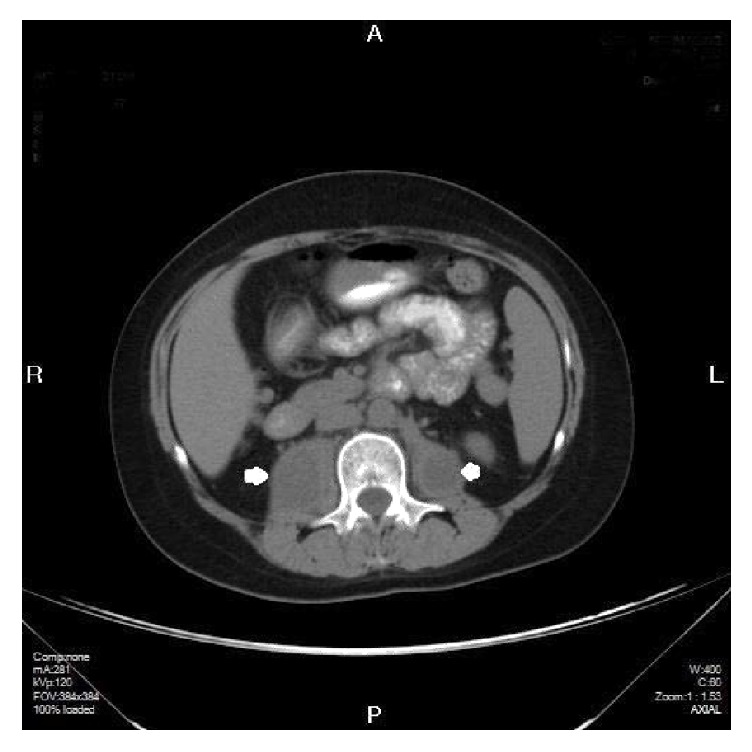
Abdominal CT scan demonstrating resolving iliopsoas fluid collections.
